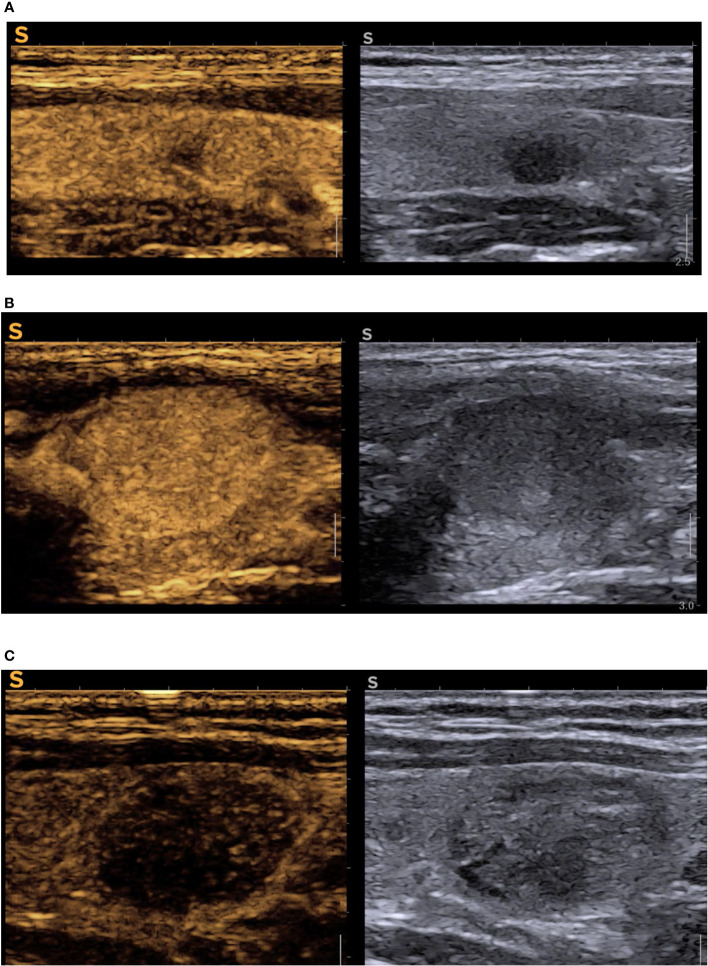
CEUS cases (A) oval, intense hypoechoic nodule with ill-defined nodules in B-mode (EU-TIRADS 5), right side of the image, reconfirmed high-risk in CEUS with inhomogeneous hypoenhancement, central enhancement defect, absent peripheral ring, in left half of the image = PTC; (B) hypoechoic subcapsular solid nodule (EU-TIRADS 4) with peripheral enhanced ring, complete iso-enhancement, no enhancement defects in CEUS (remained TIRADS 4, but it was low-risk in CEUS) = benign nodule; (C) solid, well-defined, isoechoic with some areas of hypoechogenicity which included the nodule in EU TIRADS 4, CEUS high risk due to diffuse hypoechancement (upgraded to modified-TIRADS 5) = medullary thyroid cancer. CEUS, contrast-enhanced ultrasound; TIRADS, Thyroid Imaging Reporting and Data System.
